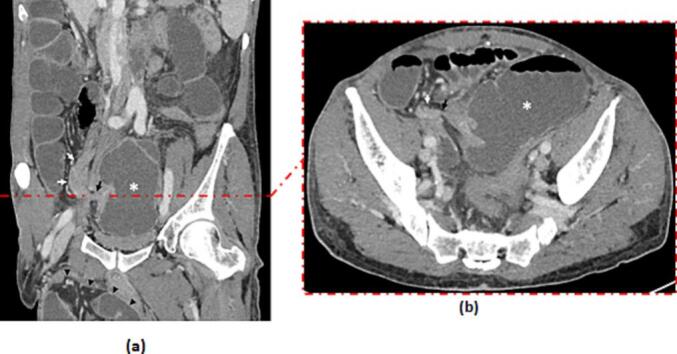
Coronal (A) and axial (B) enhanced CT images showcase a dilated caecum (white asterisk) located in the left iliac fossa and pelvis converging and tapering into a transitional point located in the right iliac fossa (black arrow) without dilation of the right colon (white arrows) nor the rest of the colonic segments. Note the right scrotal hernia (black arrow-heads) and the distension of the small bowl.
